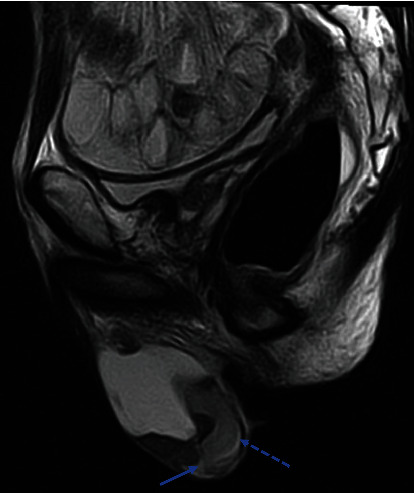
MRI T2-weighted image of the pelvis showing a 7.3 × 5.4 × 4.5 cm testicular mass consisting of a cystic solid tumor (arrow) as well as normal testicle components (dashed line arrow).
